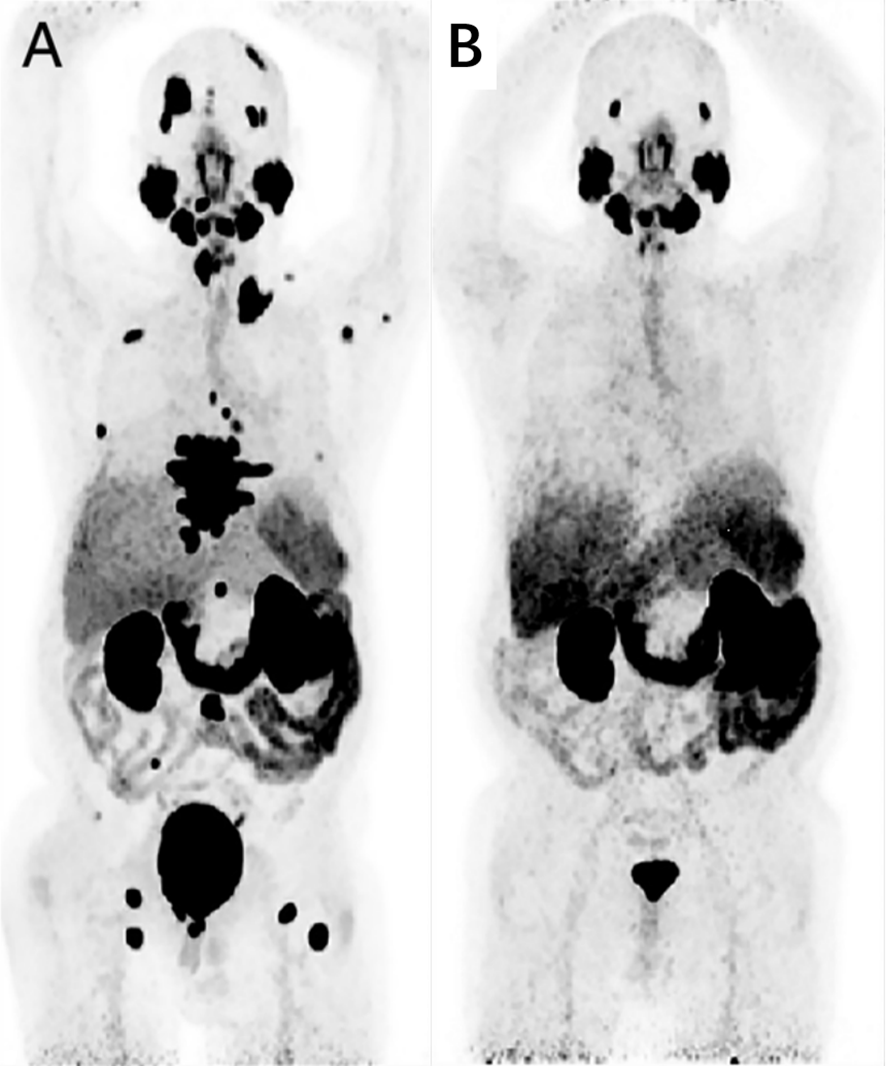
A 65-year-old man, diagnosed with prostate cancer 7 years ago during prostatectomy, experienced biochemical recurrence. A baseline ^68^Ga-PSMA-11 PET/CT imaging was conducted, and the maximum intensity projection (MIP) image revealed systemic PSMA metastases, particularly in bone metastatic lesions (A). Following 2 cycles of Lu-PSMA-617 treatment, a reassessment of treatment efficacy was performed using ^68^Ga-PSMA-11 imaging. The MIP image showed no tracer uptake in new lesions, and there was a significant reduction in tracer uptake overall (B). Adapted from Ref (81).
